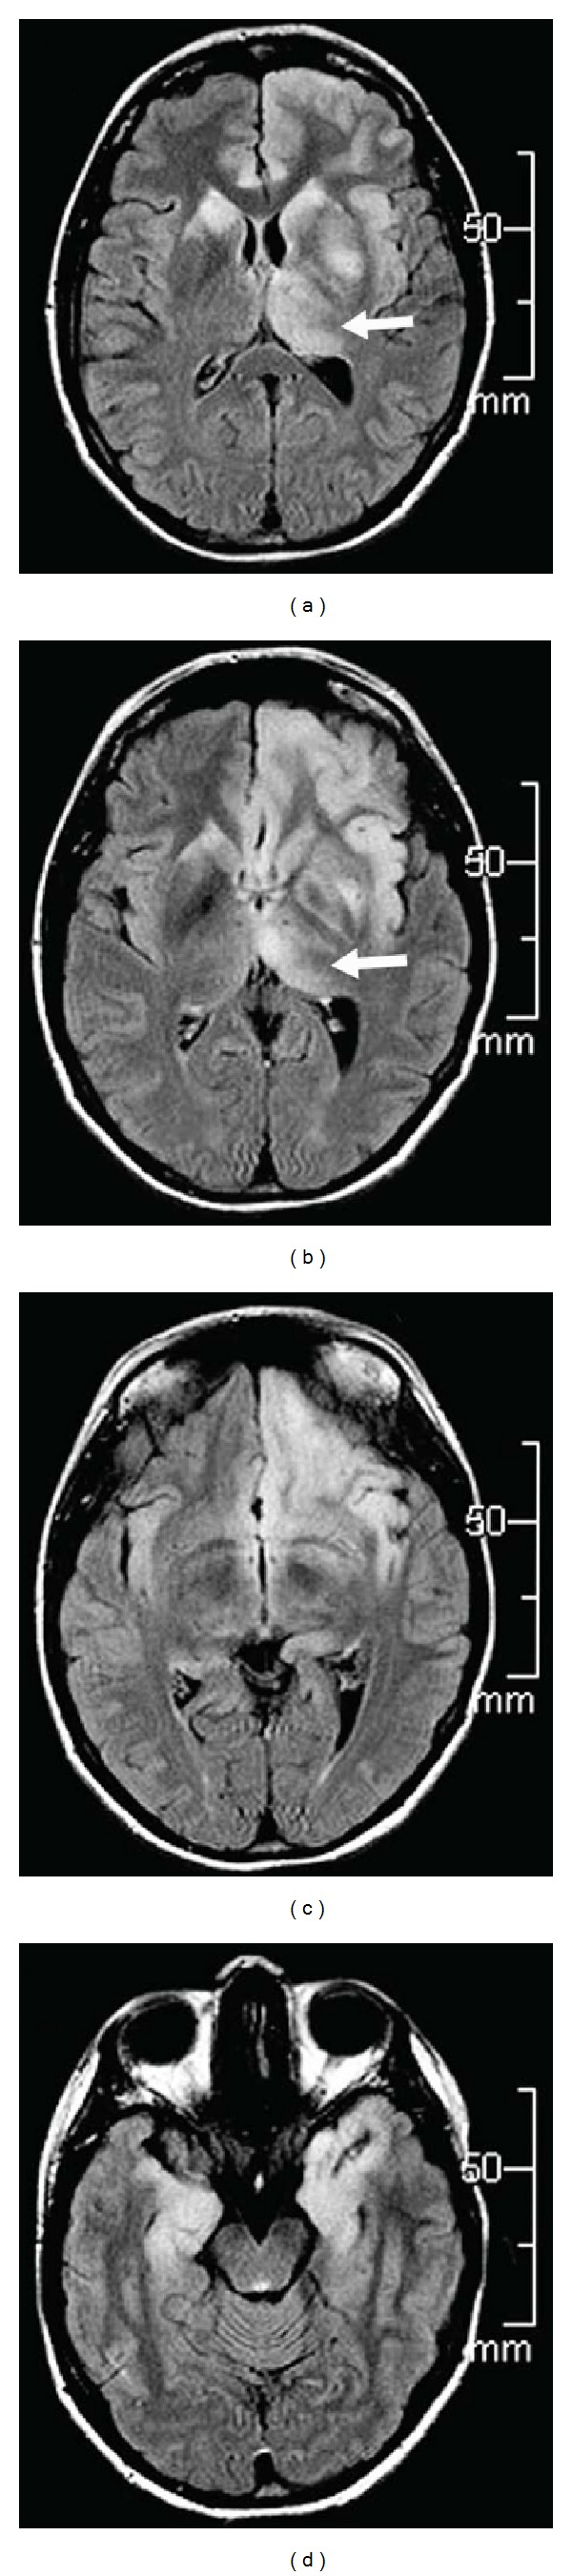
A patient with gliomatosis cerebri involving the left thalamus (arrows, a and b), the bilateral basal ganglia (a and b), the left frontal lobe (a, b, and c), and both temporal lobes (d), seen as hyperintensity on FLAIR sequences.
